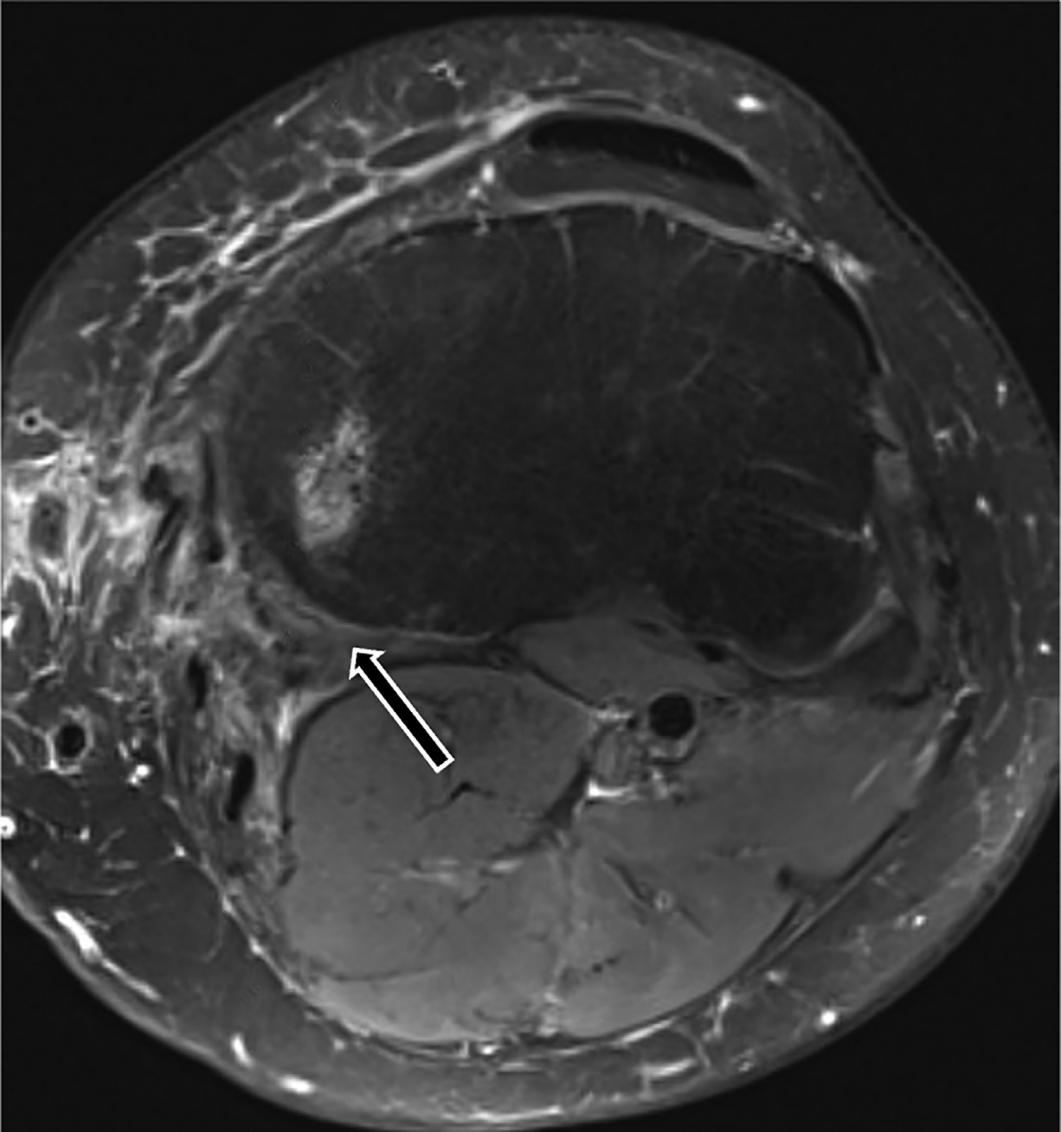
T2-weighted axial image 5 months post-repair. The arrow demonstrates the healed semimembranosus tendon at the normal posteromedial tibial plateau.
